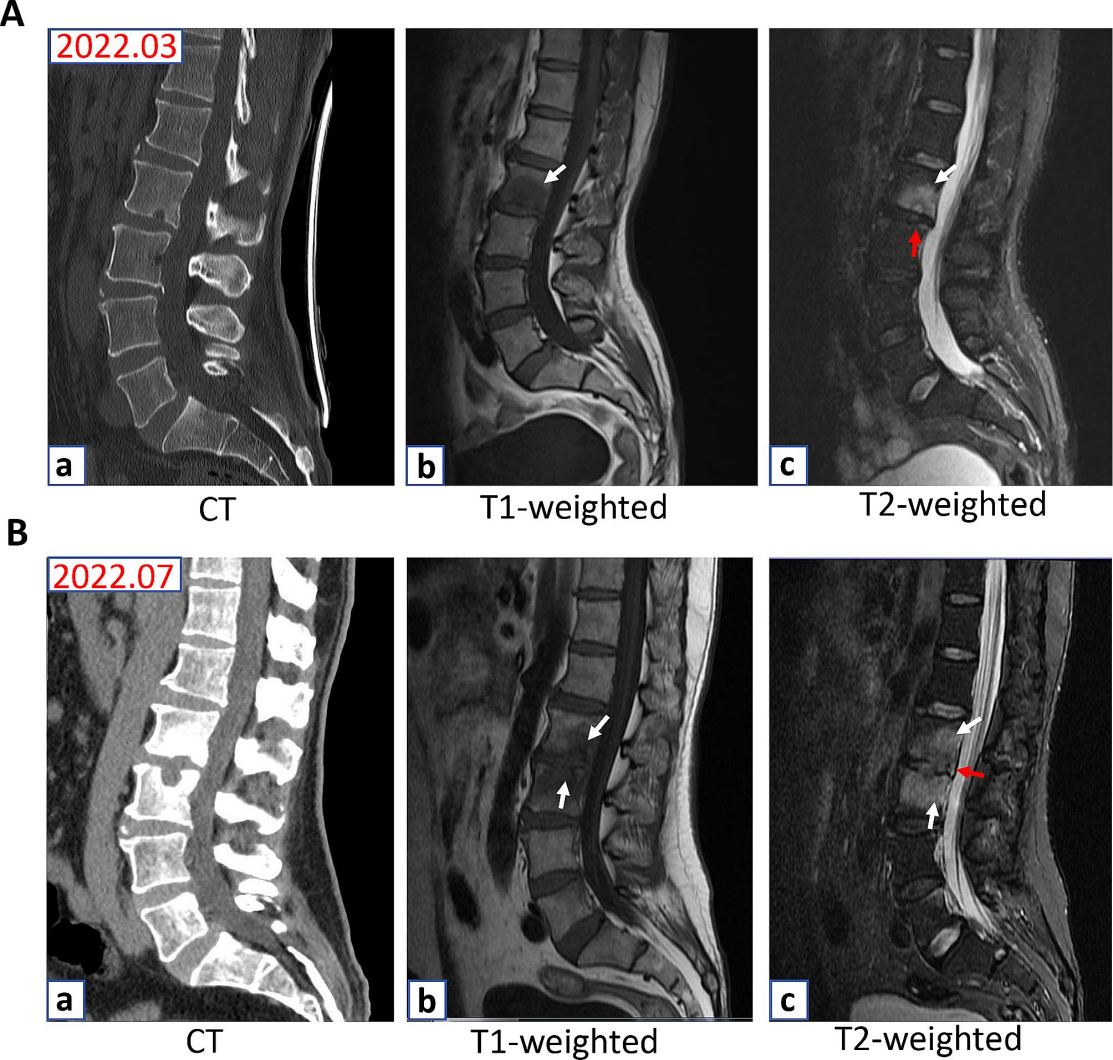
CT showed an irregular bone destruction at the L2 vertebral body (a). MRI showed irregular bone destruction at the L2 vertebral body endplate (white arrow) with a T2-weighted image (c) and a lesion with low T1 (b) and high T2 intensity (c) at the L2 vertebral body and L2–L3 intervertebral disc (red arrows) on March, 2022. Fig. 1B CT showed a significant irregular bone destruction at the L2 and L3 vertebral body (a). MRI showed irregular bone destruction at the L2 and L3 vertebral body endplate (white arrow) with a T2-weighted image (c) and a lesion with low T1 (b) and high T2 intensity (c) at the L2 and L3 vertebral body and L2–L3 intervertebral disc (red arrows) on July, 2022
